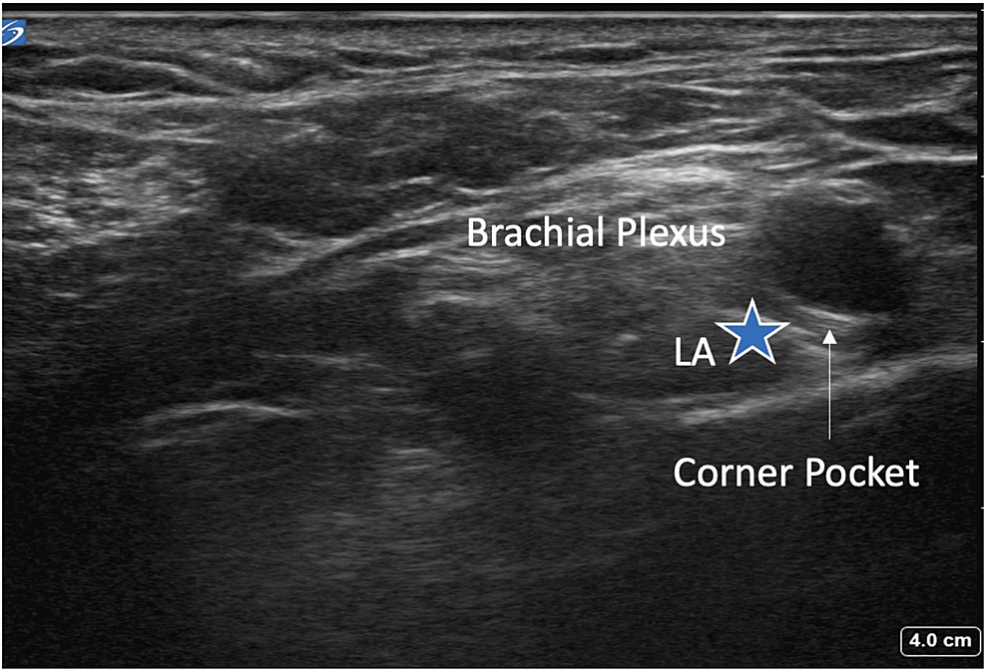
Ultrasound image of the local anesthetic deposited in the corner pocket for the supraclavicular block. LA: local anesthetic
